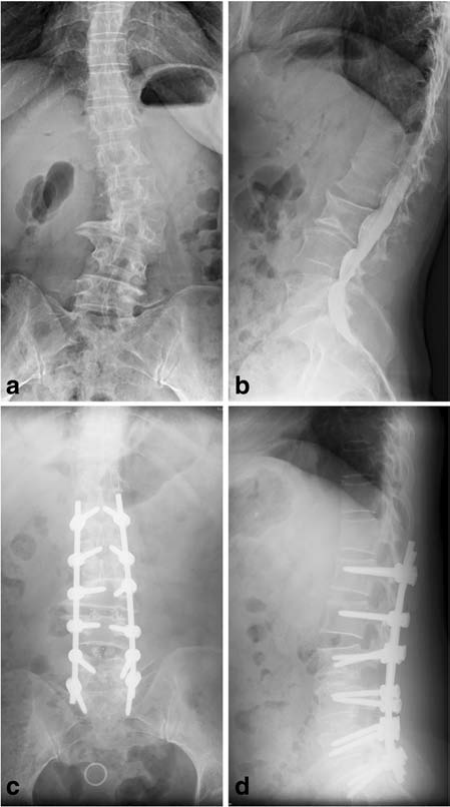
A 55 year old female patient with back pain and left leg numb pain for four months. a) preoperative radiograph showing degenerative scoliosis with 23°Cobb angle, L3/4 interval tip angle, 0.45 of W/AL. b) preoperative CT showing L4/5, L3/4, and L2/3 disc herniation. c) postoperative anteroposterior radiography showing good correction. d) postoperative lateral radiography showing satisfying lumbar lordosis
